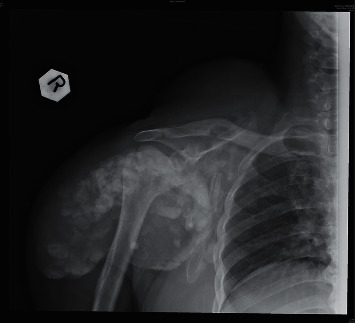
Radiograph of the right shoulder before teriparatide therapy.
